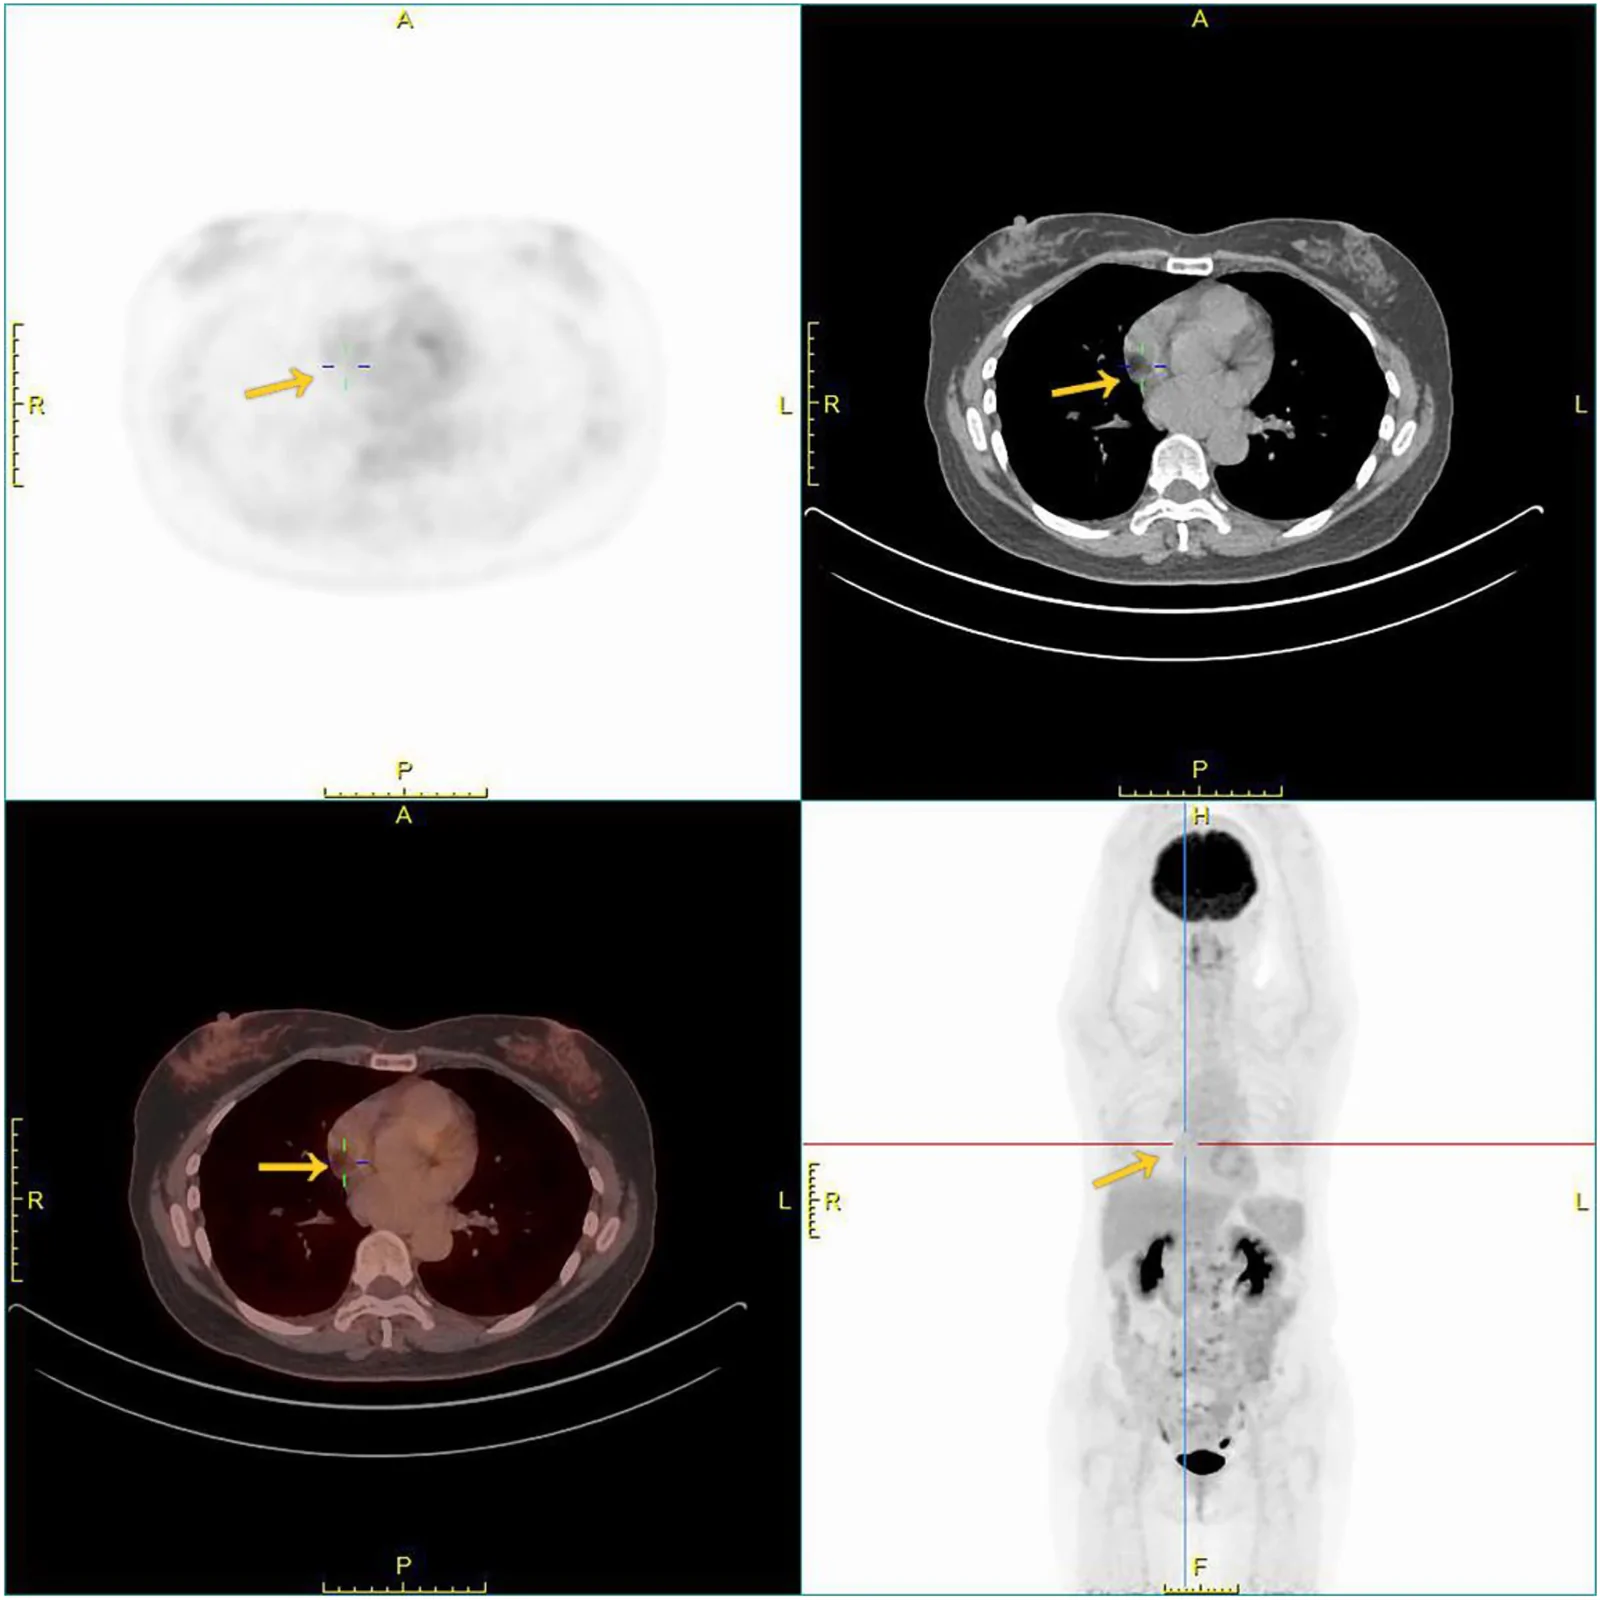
The PET/CT fusion image demonstrated reduced FDG uptake within the lesion (SUVmax = 1.3), in contrast to the physiological uptake observed in the surrounding myocardium. The arrow indicates the location of the mass. FDG = ^18^F-fluorodeoxyglucose, PET/CT = positron emission tomography/computed tomography, SUVmax = maximum standardized uptake value.
